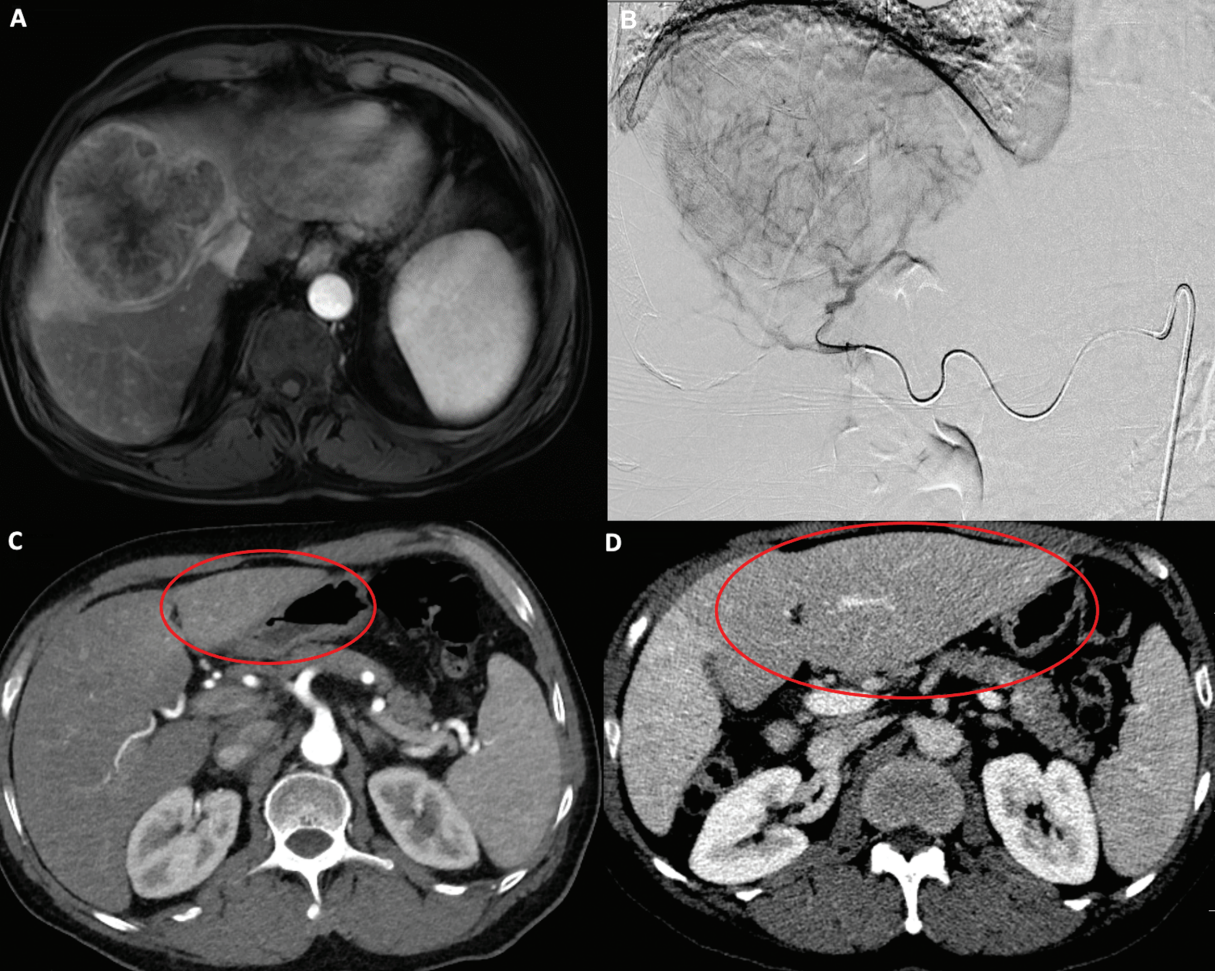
(A) Contrast-enhanced MRI demonstrated a large 6.9 cm segment V and VIII right hepatic lobe HCC. (B) Intraprocedural digital subtraction angiogram demonstrating hypervascular HCC mass to be treated with right hepatic artery radiation lobectomy. (C) The contralateral left hepatic lobe (FLR) is circled prior to TARE. (D) Left hepatic lobe, FLR, is circled 9 months after TARE and demonstrates compensatory hypertrophy.
